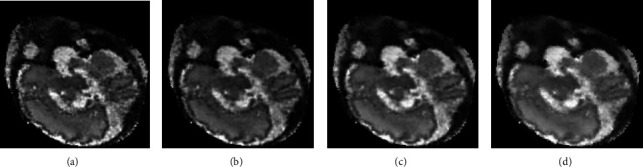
Reconstructed results of the MRI data with different structure sensitivities *α*: (a) *α* = 0.2, (b) *α* = 0.3, (c) *α* = 0.4, and (d) *α* = 0.5.
